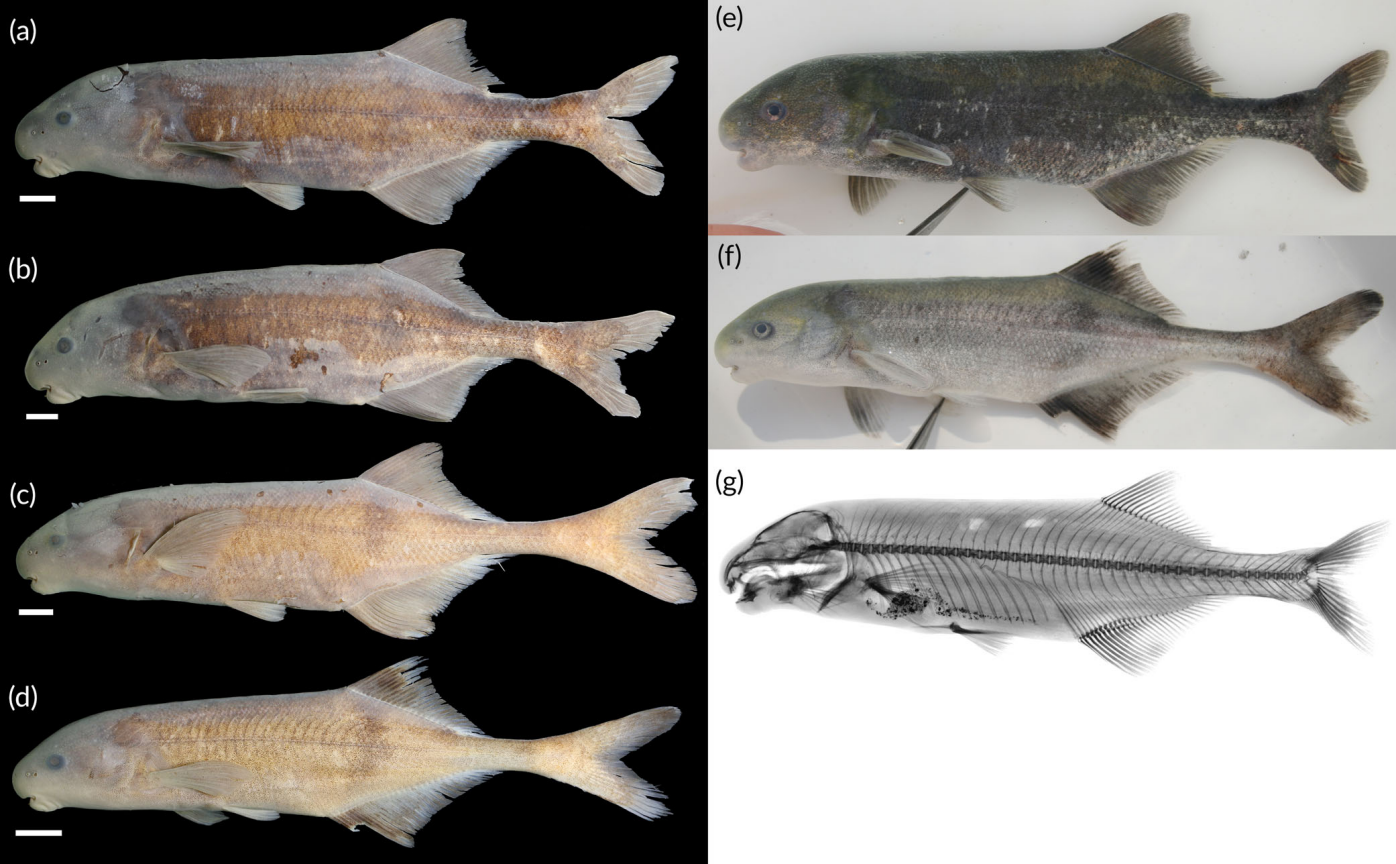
(a) Holotype of *Heteromormyrus dolichorhynchus* sp. nov. (SAIAB 85219), female 151.0 mm standard length (SL), from the Kwanza River; (b) paratype of *H. dolichorhynchus* sp. nov. (SAIAB 85219), male 155.0 mm SL, from the Kwanza River; (c) *H. dolichorhynchus* sp. nov. (SAIAB 84645), male 151.0 mm SL, from the Kwanza River; (d) *H. dolichorhynchus* sp. nov. (SAIAB 84645), female 117.0 mm SL, from the Kwanza River; (e, f) live pictures of *H. dolichorhynchus* sp. nov.; (g) X‐ray radiograph of holotype. Scale bar =1 cm.
